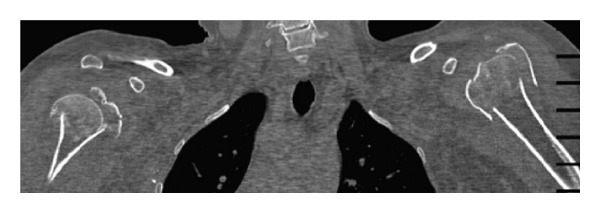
Coronal view of the CT of both shoulders demonstrating four-part proximal humeral fractures bilaterally.
